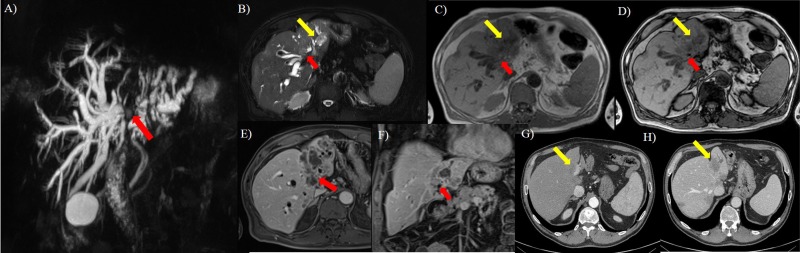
Sixty-three years old man with colorectal cancer. A, MIP image. Interruption of confluence of the right and left hepatic ducts with biliary tree dilation. In B (axial T2-W HASTE FS) it is evident the presence of peri-intraductal ductal hyperintense tissue at confluence of the right and left hepatic ducts (red arrow) and in peri- left hepatic ducts (yellow arrow), that appear hypointense (red and yellow arrow) in C (axial T1-W in phase) and D (axial T1-W out phase), with progressive contrast enhancement in E (arterial phase) and D (portal phase). On CT study in F (arterial phase there is no evidence of lesion, while in portal phase (G) the tumor (yellow arrow) appears as a hypodense hepatic lesion.
